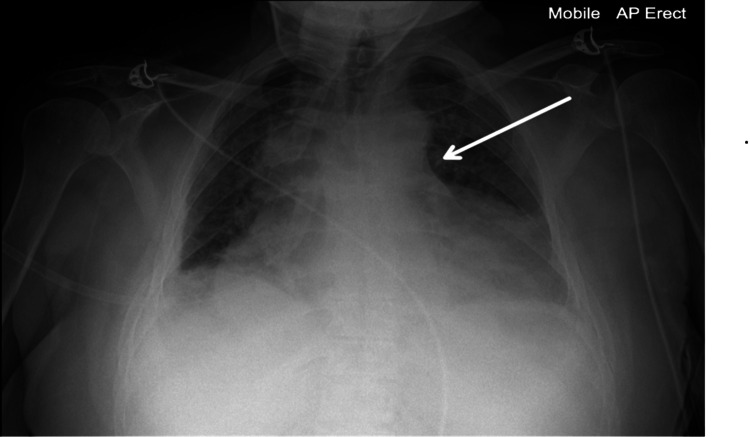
Chest X-ray showing a bulky mediastinum with poorly defined heart borders and bilateral pleural effusions The white arrow points to the widened mediastinal borders.
